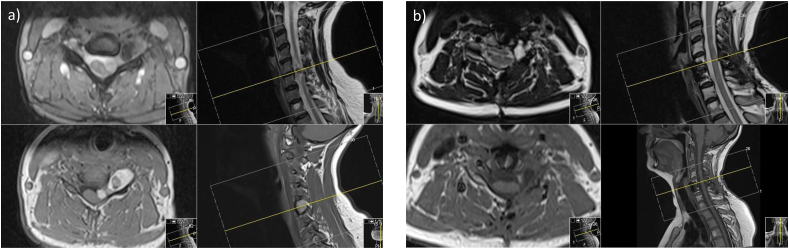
A) Pre-operative magnetic resonance imaging (MRI) in T2 (superior plates) and T1 (inferior plates) weighted imaging sequences. Axial (left) and Sagittal (right) sequences presented. The left-sided dumbbell shaped lesion is visualised extending through the intervertebral foramen with both intra-dural intra-canalicular and extra-dural extra-canalicular elements. The T1 weighted sequence demonstrates the characteristic ‘target’ sign with central hypo-intensity with circumferential peripheral enhancement. b) Post-operative MRI in similar configuration demonstrating near total excision of the lesion. A small focus of contrast-enhancement intra-dural and intra-canalicular is visualised representing possible residual remnant.
